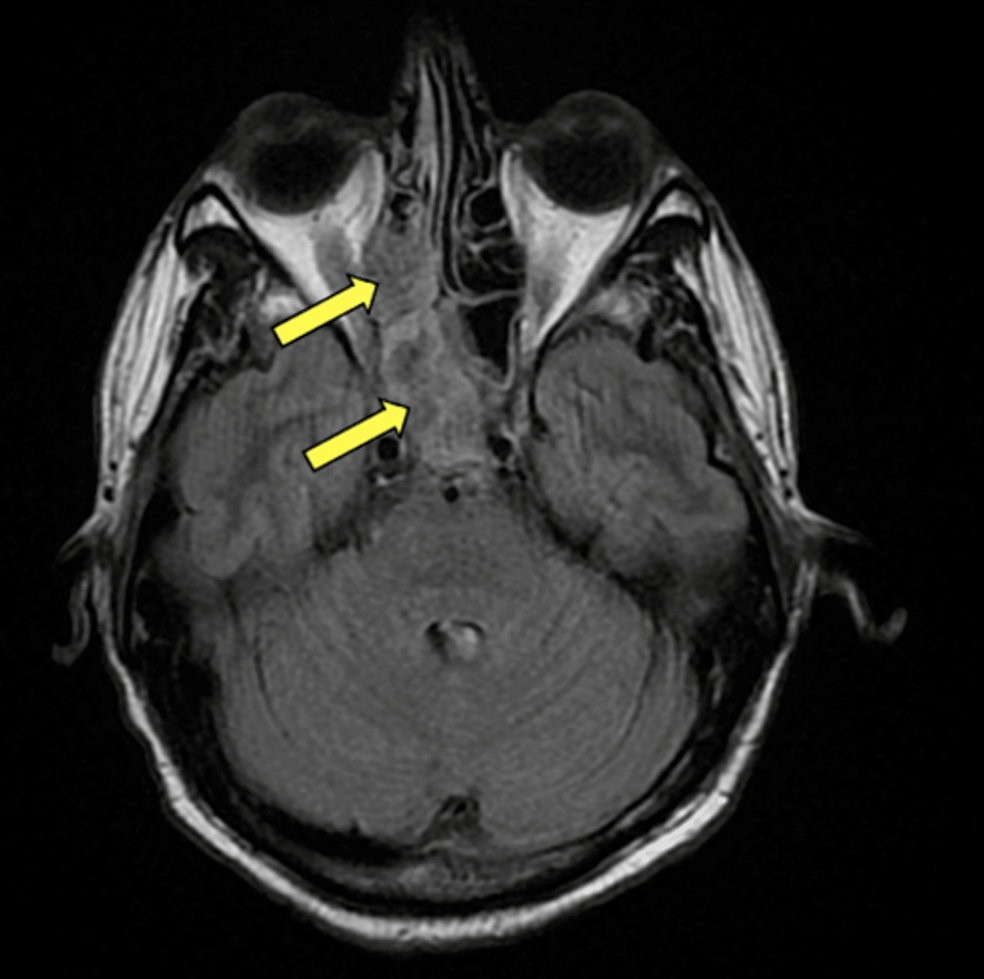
MRI orbit face and neck with contrast Findings show right sphenoethmoidal fungal sinusitis (yellow arrows). There is infection and an 8 mm sinonasal polyp in the right sphenoethmoidal recess/posterior superior nasal canal.
